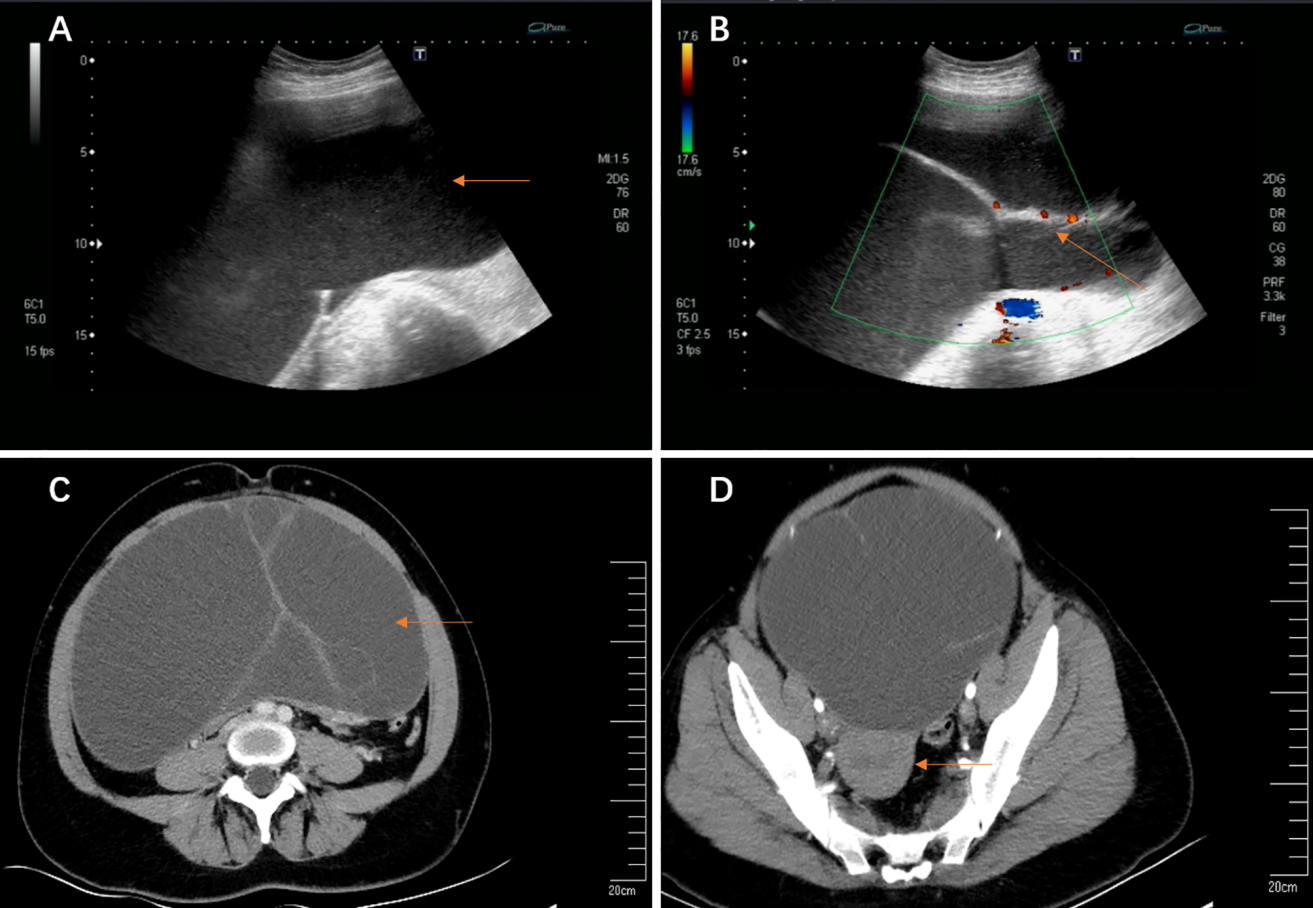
(A, B) Transvaginal ultrasound imaging. (A) A giant cyst in the abdominal and pelvic cavity (63.0 cm × 44.0 cm × 13.4 cm); (B) The blood flow signal detected at the separation; (C, D) Abdomen enhanced CT imaging. (C) A giant cyst with septums; (D) The uterus was pushed to the back of the pelvis by a giant cyst.
